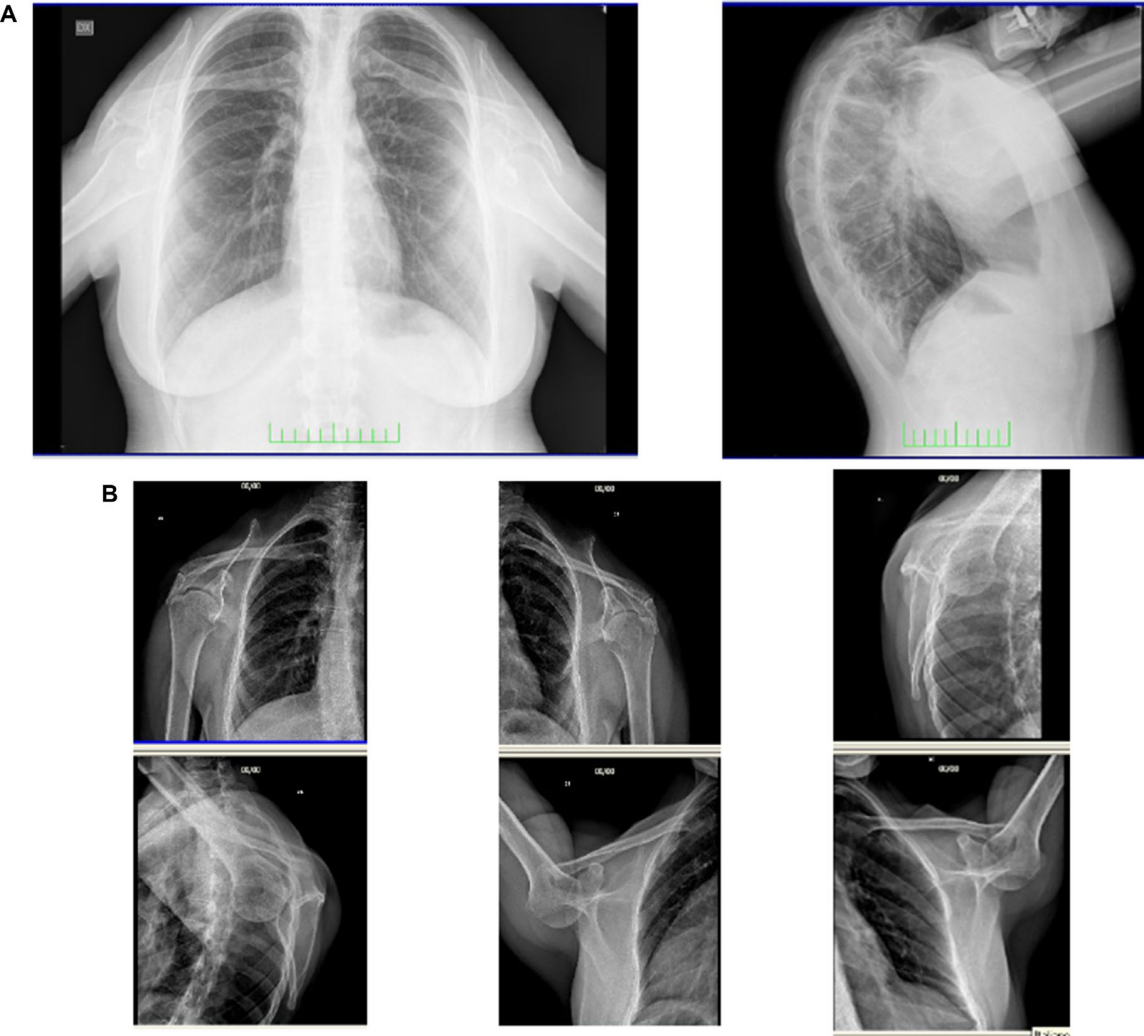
Frontal and lateral radiograph of the patient (A). Small, low, and laterally set scapulae with the glenohumeral joints lower than normal and down-turned clavicles are shown (B).
